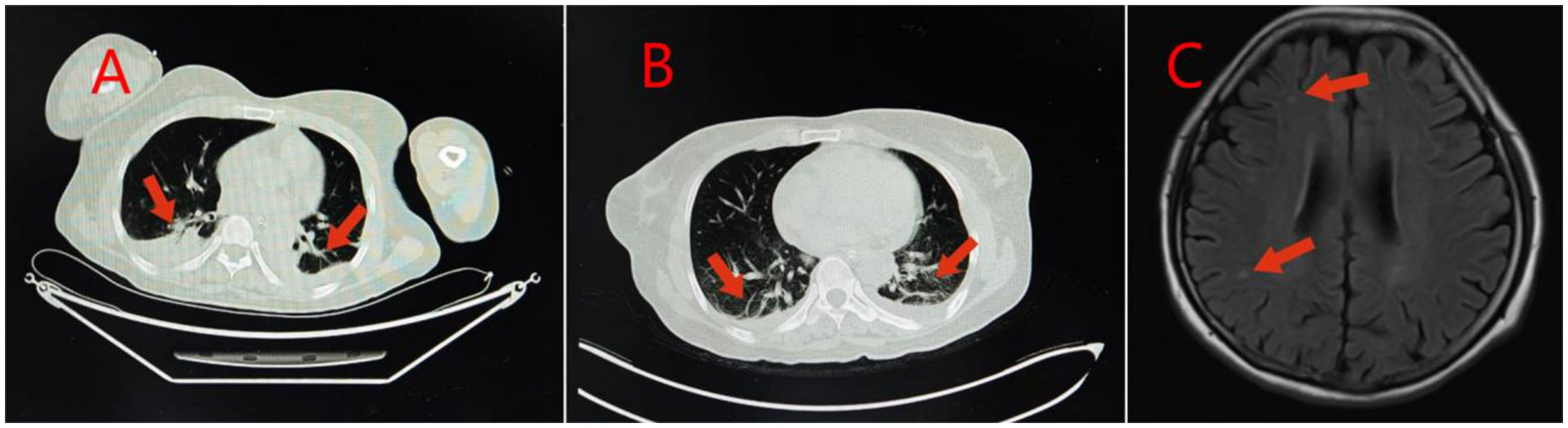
Imaging findings in Case 2: Panel (A) Lung CT shows inflammation or hypostatic changes in both lungs, and a small amount of pleural effusion in both pleural cavities; Panel (B) Reexamination of lung CT shows inflammation, fibrous foci, and hypostatic changes in both lungs, and a small amount of pleural effusion in both pleural cavities; Panel (C) Brain MRI shows a small amount of ischemic degenerative foci in the white matter of both cerebral hemispheres.
